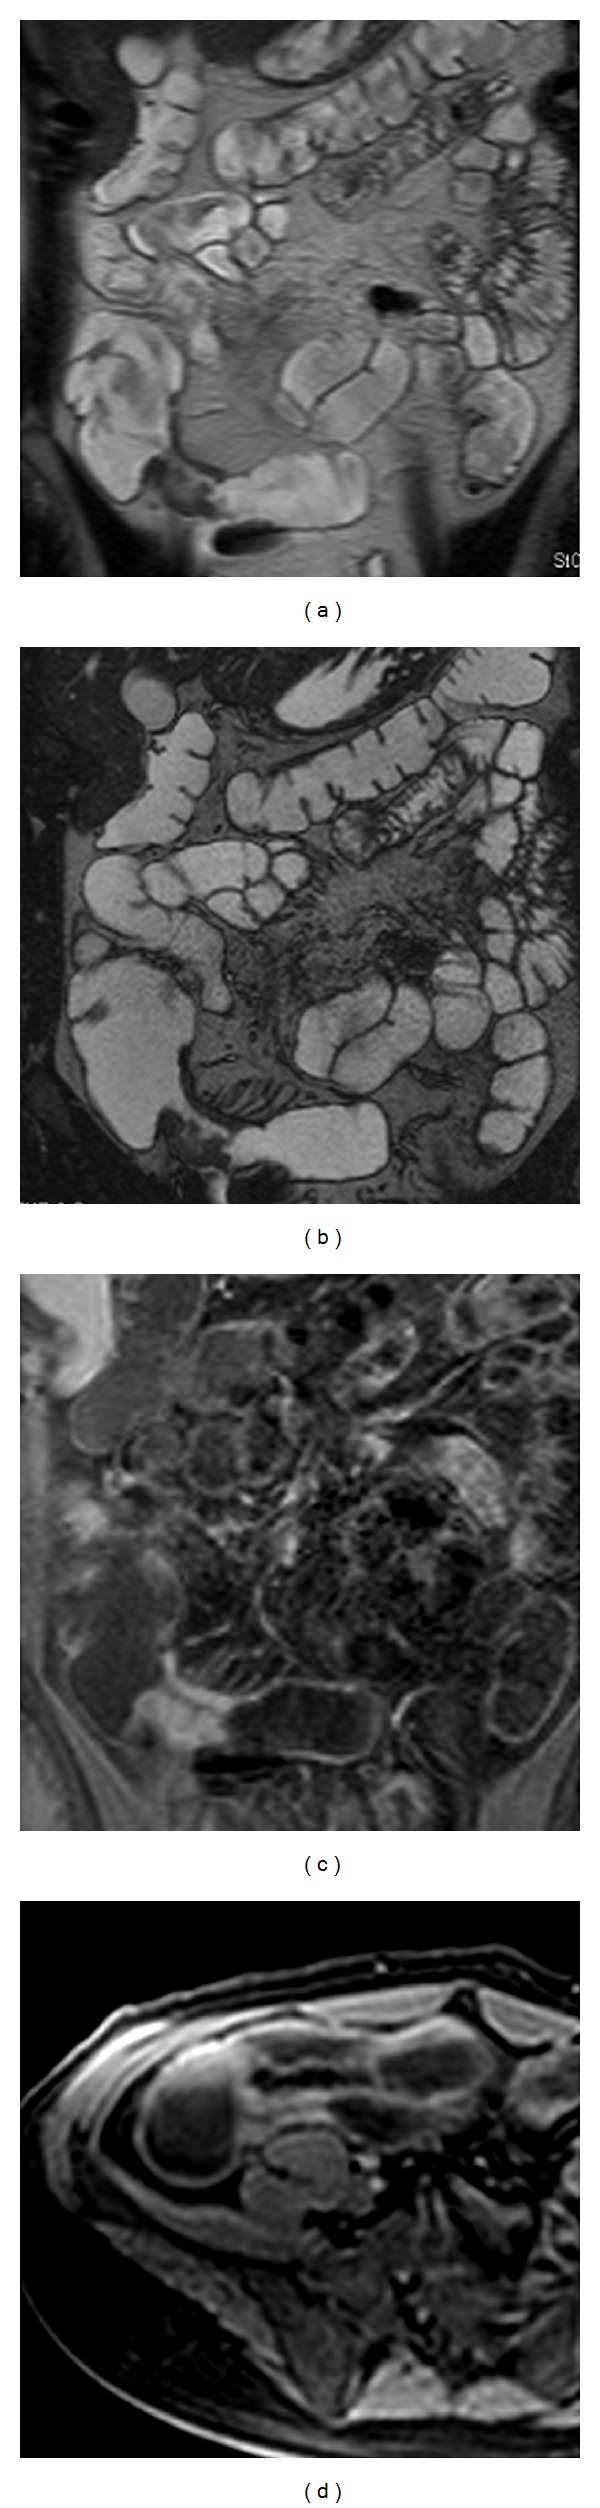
(a) MR enterography, T2 weighted sequence (HASTE, half-Fourier axial single-shot fast spin-echo): relapse of segmental disease associated with segmental wall thickening at the site of previous ileocolic anastomosis; (b) MR enterography T2 weighted sequence (TrueFISP, true fast imaging with steady-state free precession) delineated profiles of segmental wall thickening and mild *comb* sign; (c-d) MR enterography, T1-weighted sequence (VIBE, volumetric interpolated breath-hold examination): postcontrast homogeneous enhancement of the intestinal wall suggesting a fibrotic lesion.
